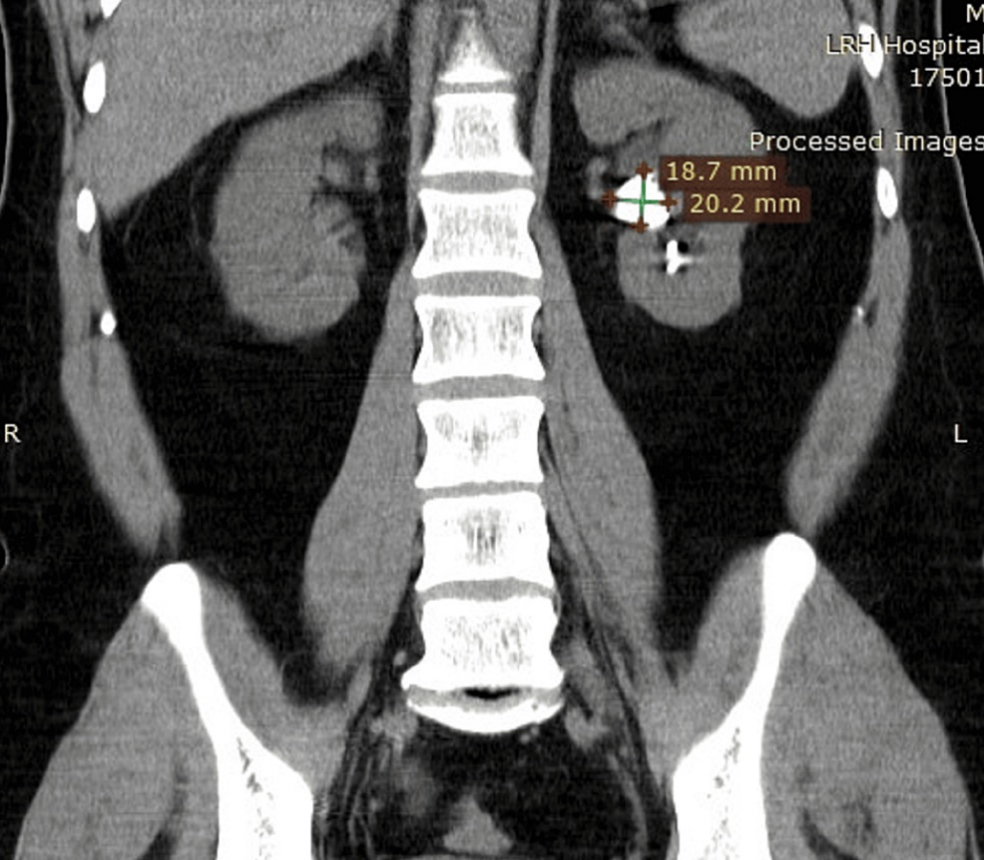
Preoperative CT KUB image showing a large left kidney stone. CT KUB = computed tomography of the kidneys, ureters, and bladder
